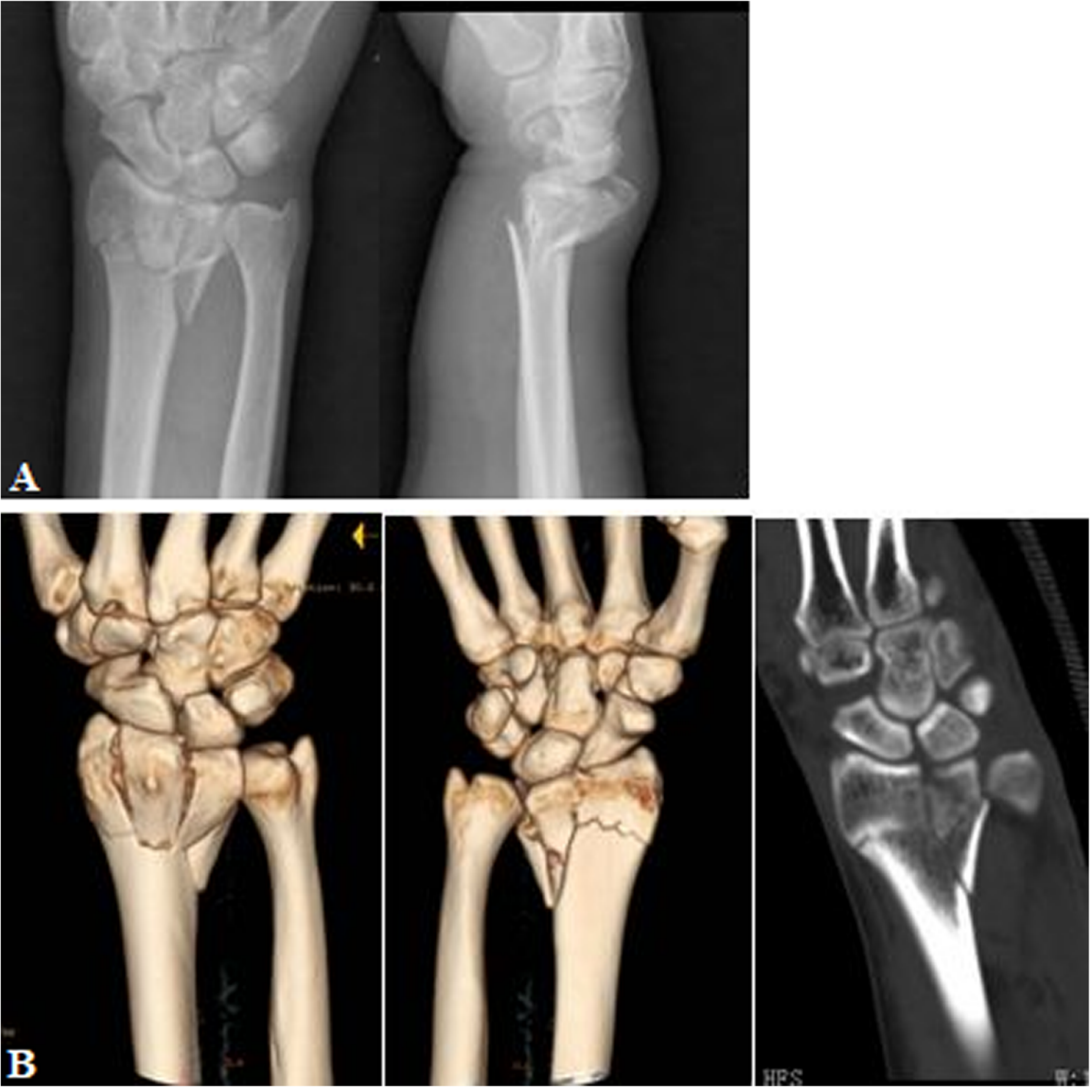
PA X-ray (a) and CT scan (b) of combined radius column fracture
